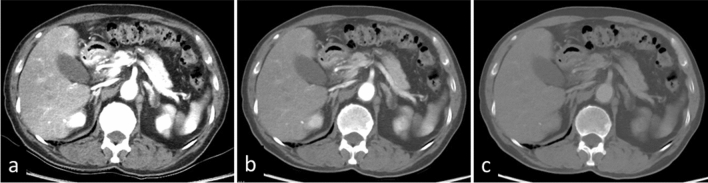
Virtual monochromatic images obtained at 40 (a), 70 (b), and 140 keV (c) (window level/width; 30/580 HU). On dual-energy CT scans, a monochromatic image, looking as if it had been acquired with single energy (keV), can be synthesized arbitrarily. The CT attenuation number on approximately 65–70 keV virtual monochromatic images is equivalent to single-energy CT scans acquired at 120 kV. The iodine contrast increases as the energy level decreases
